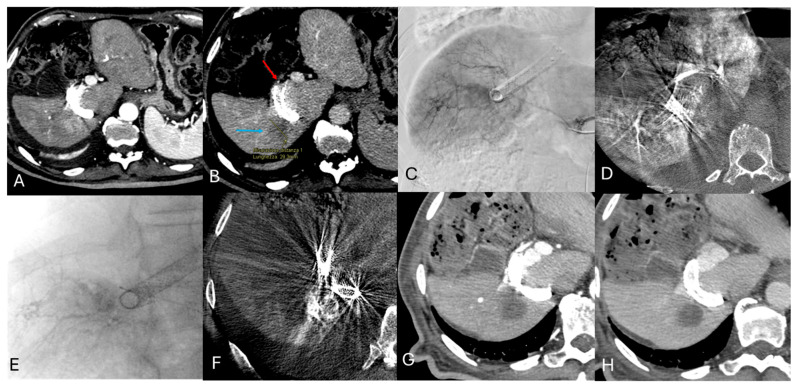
76 yo, male, Child B8 cirrhosis with a 3 cm HCC nodule, unfit for ablation for its adjacency to a Transjugular Intrahepatic Portosystemic Shunt (TIPS) procedure. (A) Arterial phase shows 3 cm hypervascular HCC nodule (VIIs-VIs) with *wash-in* features and (B) early *wash-out* in nephrogenic phase (blue arrow); the nodule is posterior to the TIPS (red arrow). (C) Pre-operative work-up: non-selective DSA is used to assess the hepatic lobar arterial anatomy and identify which lobar/segmental branches supply tumor feeders. (D) Pre-operative CBCT arterial phase. (E) Selective angiography via coaxial microcatheter confirms tumor enhancement. In the 2 phases of DSM-TACE, 4 mL Embocept + Epirubicin + contrast, and the remaining 3.5 mL Emobocept + contrast, were injected until stop flow. (F) Immediate post-procedural non-enhanced CBCT demonstrates that nodule is totally covered and opacified with embolics. (G,H) Arterial and venous phase of 1-month CT scan shows lesion’s complete response (CR) with a single superselctive DSM-TACE session.
